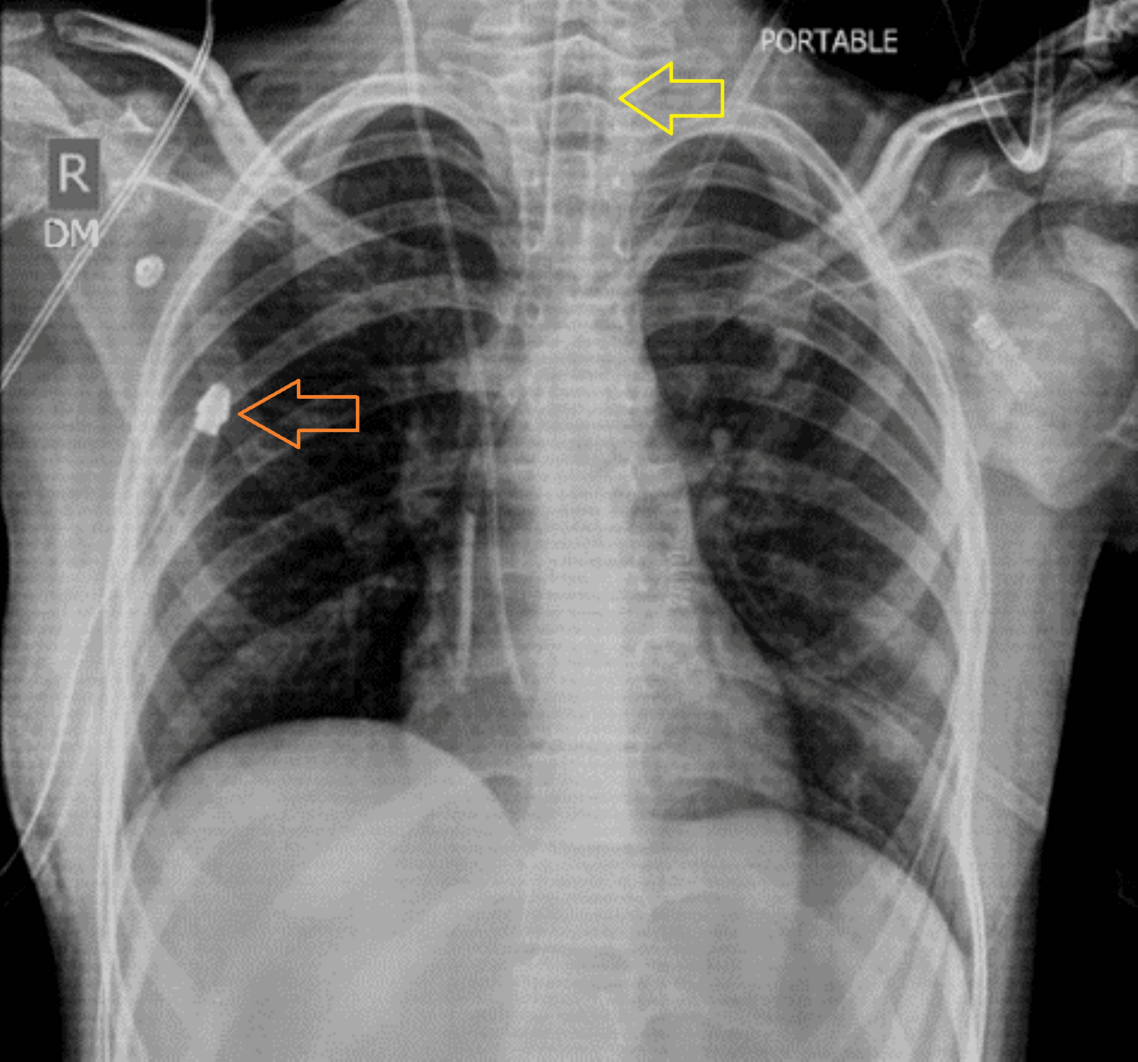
Chest X-ray on the eighth day of hospitalization, six hours after clamping the chest tube, with the chest tube (orange arrow) and endotracheal tube (yellow arrow) in place
